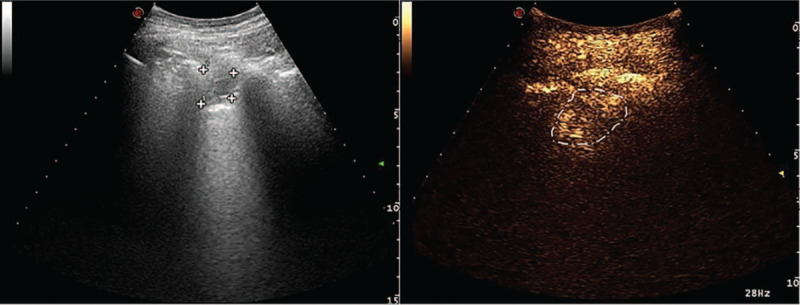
Transthoracic lung ultrasound showed hypoechoic subpleural lesion in her left lower lobe. The lesion was inhomogeneous with clear margins, ∼24 mm ^∗^ 22 mm in size. The contrast enhanced ultrasound presented a small amount of perfusion in periphery, but no perfusion in the center of the lesion. On ultrasound and contrast enhanced ultrasound imaging, the lesion was indicative of a chronic inflammatory lesion.
